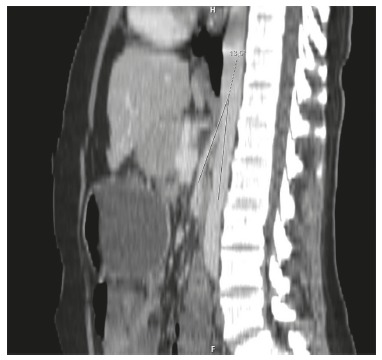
CT of the abdomen showing reduced angle between the superior mesenteric
